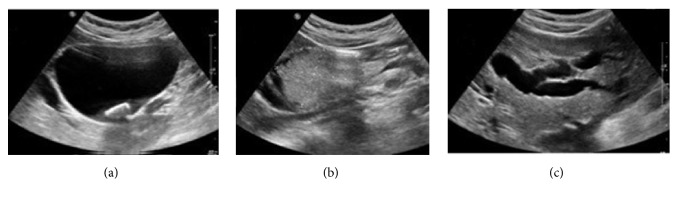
Ultrasound transverse sections. (a) Cystic mass with a gallstone independent of the gallbladder. (b) Intracystic tissue bud. (c) Dilatation of the intrahepatic bile ducts.
